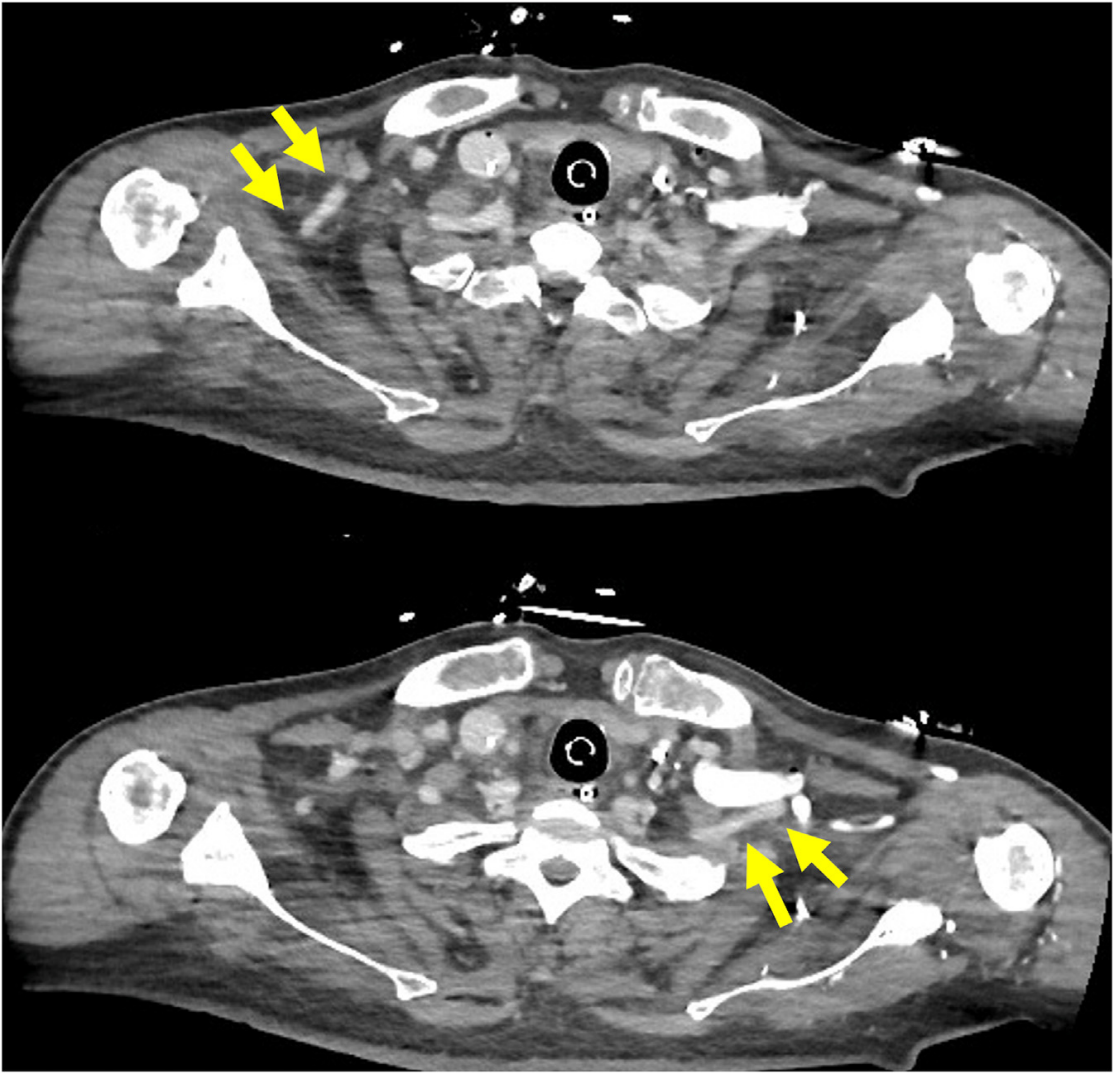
Preoperative computed tomography (*CT*) shows bilateral axillary artery measuring less than 5.5 mm (*yellow arrows*).
